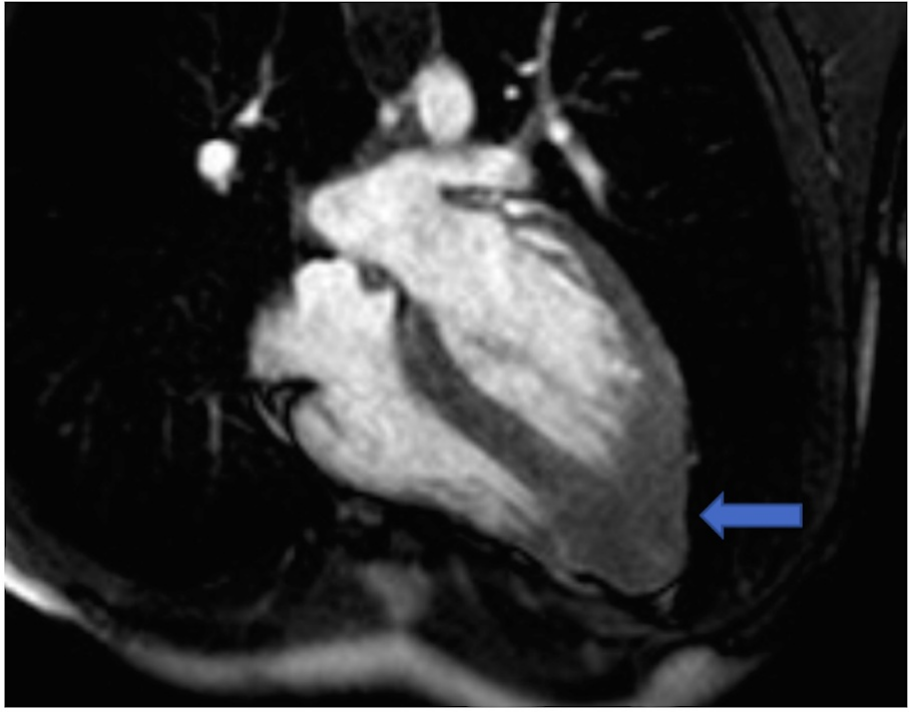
Cardiac MRI: asymmetrical left ventricular hypertrophy characterized by basal, mid inferoseptal, and apical thickness (arrow) MRI - magnetic resonance imaging.
